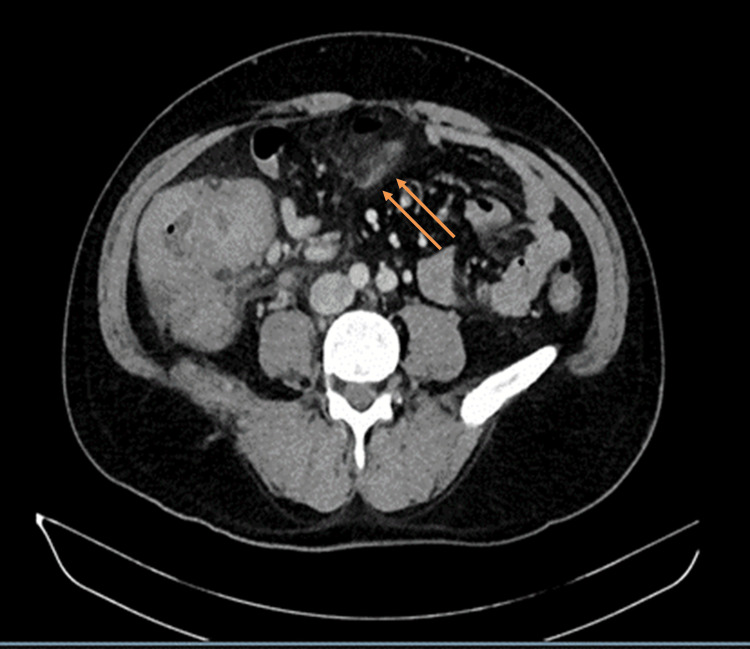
Contrast-enhanced CT scan of the abdomen showing right-sided colonic mass suggestive of malignancy Axial CT image demonstrating circumferential thickening of the cecum and ascending colon (white arrow) with luminal narrowing, mesocolic fat stranding, and multiple small regional lymph nodes (short-axis <14 mm), findings most suggestive of a right-sided colonic malignancy CT: computed tomography
